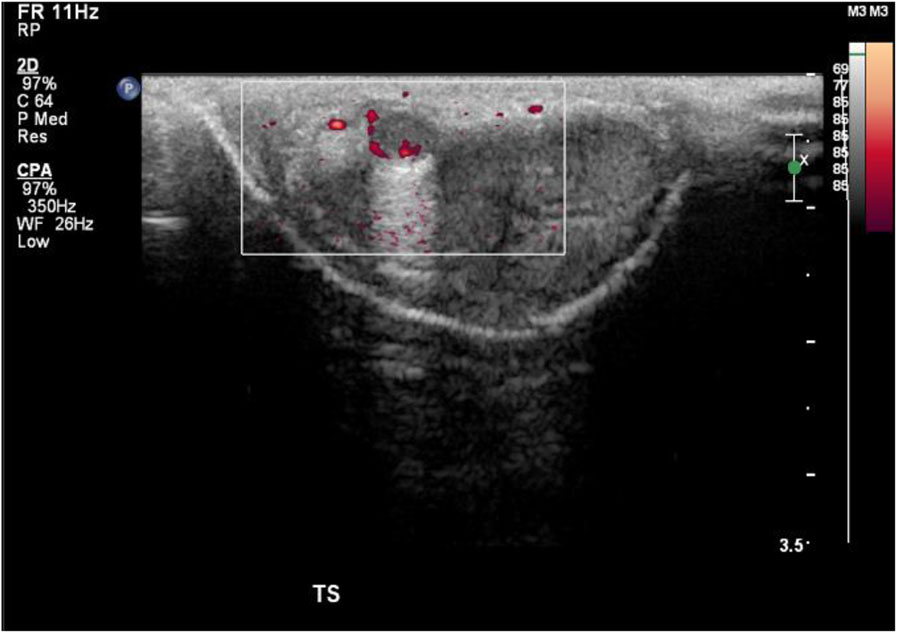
Ultrasound image (longitudinal view) of penile nodules with power doppler interrogation. Ultrasound image (longitudinal view) with power doppler interrogation reveals the presence of internal vascularity within the nodule
